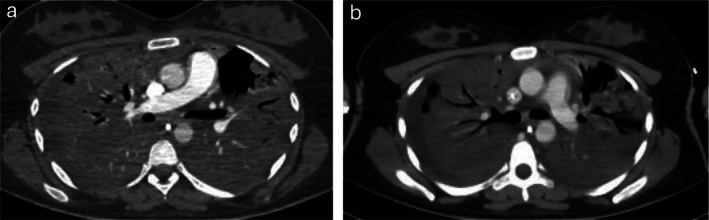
Axial Chest CT scan with contrast (mediastinal window). The pulmonary trunk, right and left main pulmonary arteries, and their lobar branches are well opacified, with no evidence of intraluminal filling defects.
